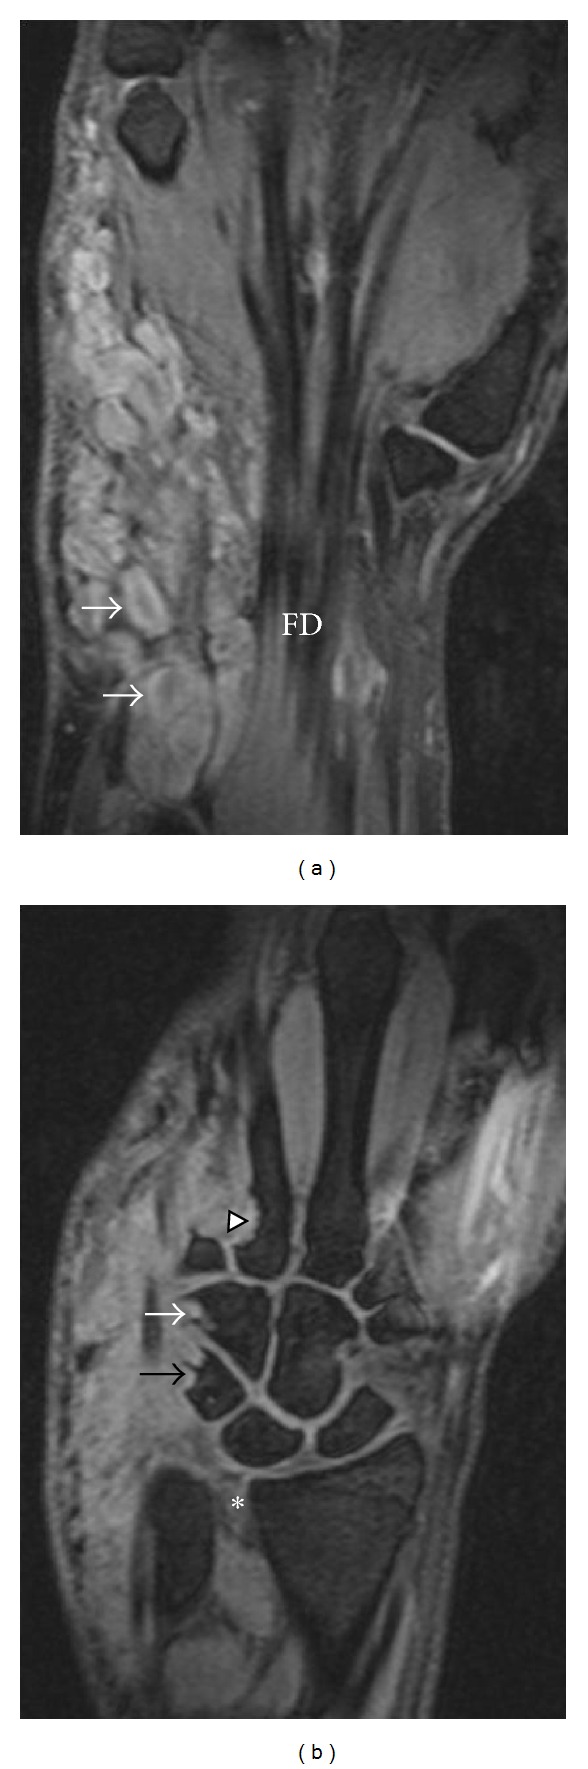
Coronal T2-weighted wrist MRI with fat suppression—(3a) anterior section: multilobulated mass abutting flexor digitorum longus (FD). Target signs (white arrows). (3b) Posterior section: remodeling of triquetrum (black arrow), hamate (white arrow), and proximal metacarpal (arrowhead). *distal radioulnar joint widening.
